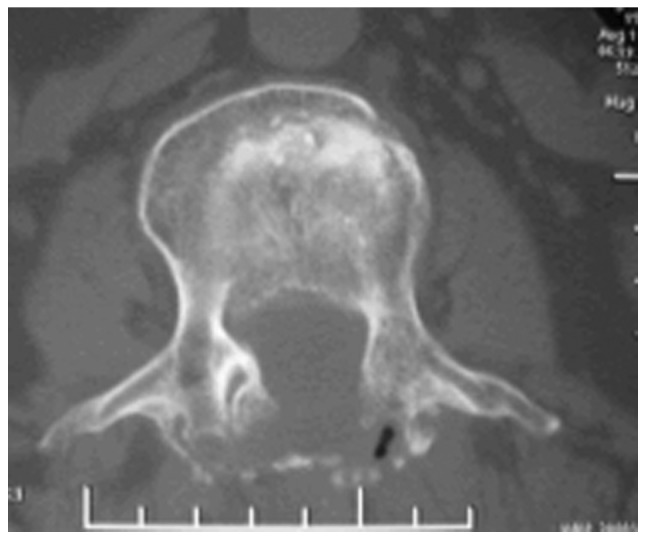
CT scanning image following removal of the internal fixation at one year after surgery of a 45-year-old male.
